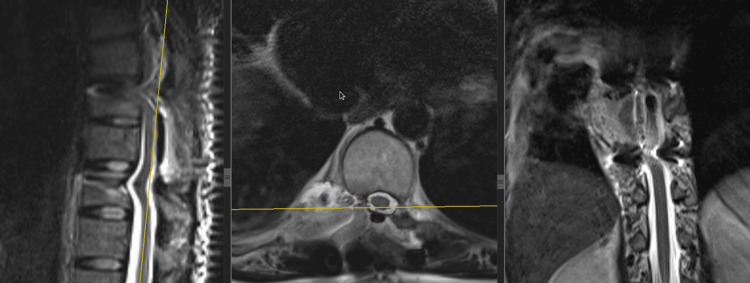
Follow-up MRI of the thoracic spine two years after surgery, including sagittal, axial, and coronal T2-weighted sequences, showing no evidence of local tumor recurrence.
